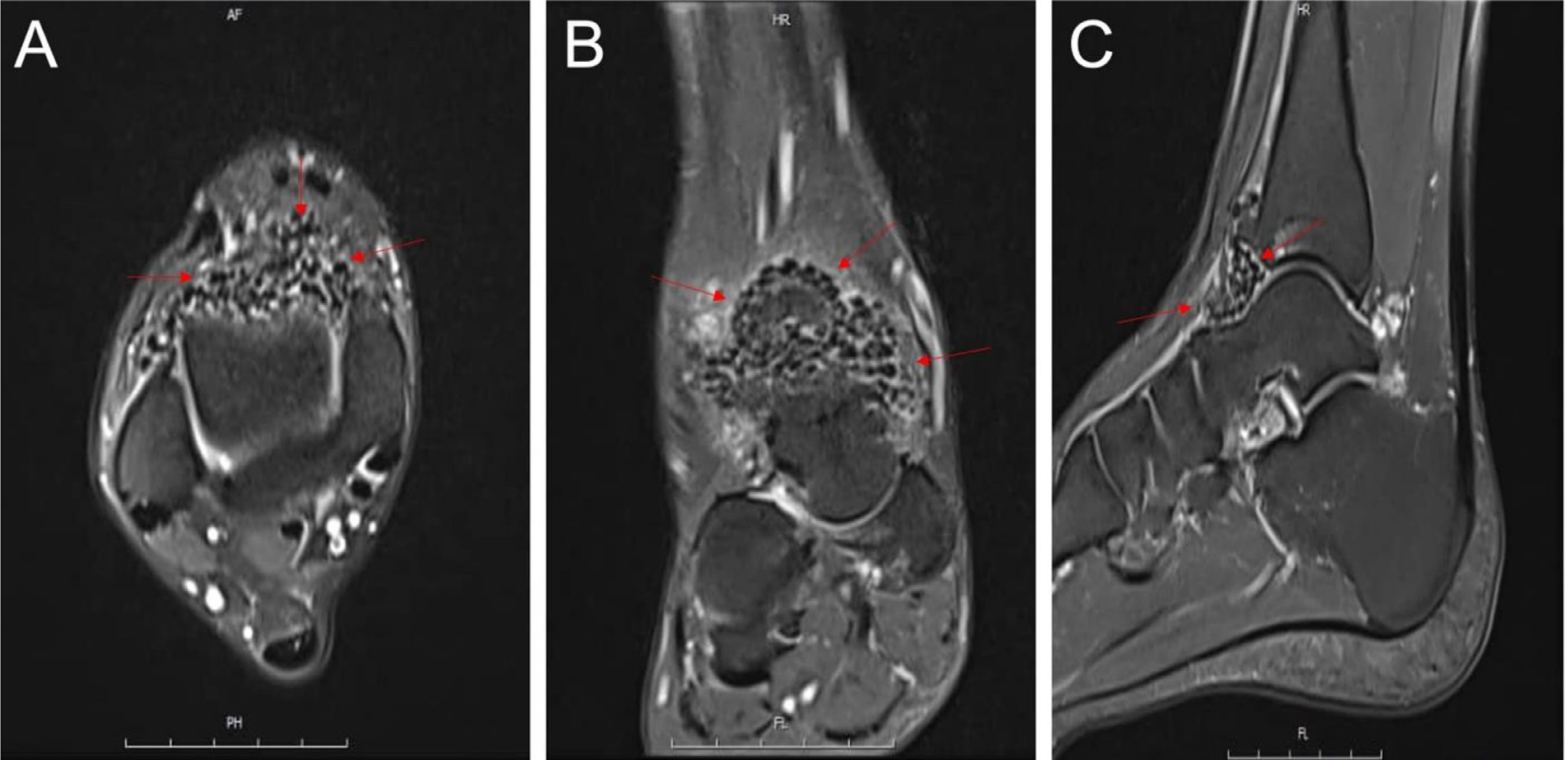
Preoperative MRI of the right foot and ankle showing synovial chondromatosis lesions (loose bodies) in the anterior part of the ankle, indicated by red arrows. The loose bodies appear as low-signal intensity structures within the joint space on MRI, consistent with calcified or ossified tissue. (A) Axial view, (B) Coronal view, (C) Sagittal view. These findings represent intra-articular fragments characteristic of synovial chondromatosis.
